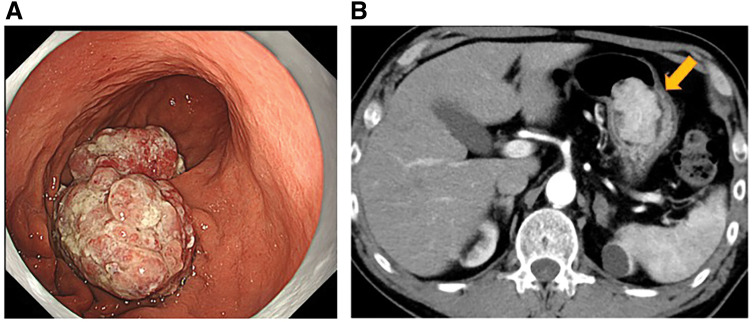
Preoperative findings. (A) Upper gastrointestinal endoscopy showed the lesions extended beyond the submucosal layer. (B) Contrast-enhanced CT scan showed a 30-mm tumor in the gastric body. The yellow arrow indicates the tumor.
